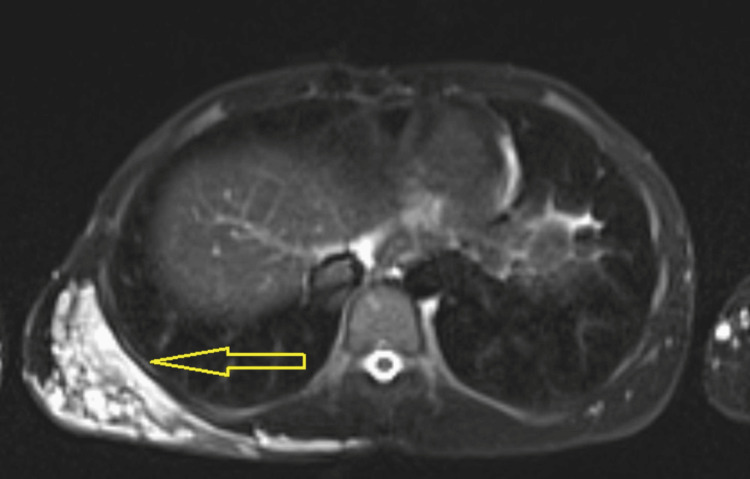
Axial T2-weighted MRI of the chest, demonstrating a large, hyperintense lesion in the right posterior chest wall with multiple dilated and tortuous vascular channels MRI: magnetic resonance imaging
